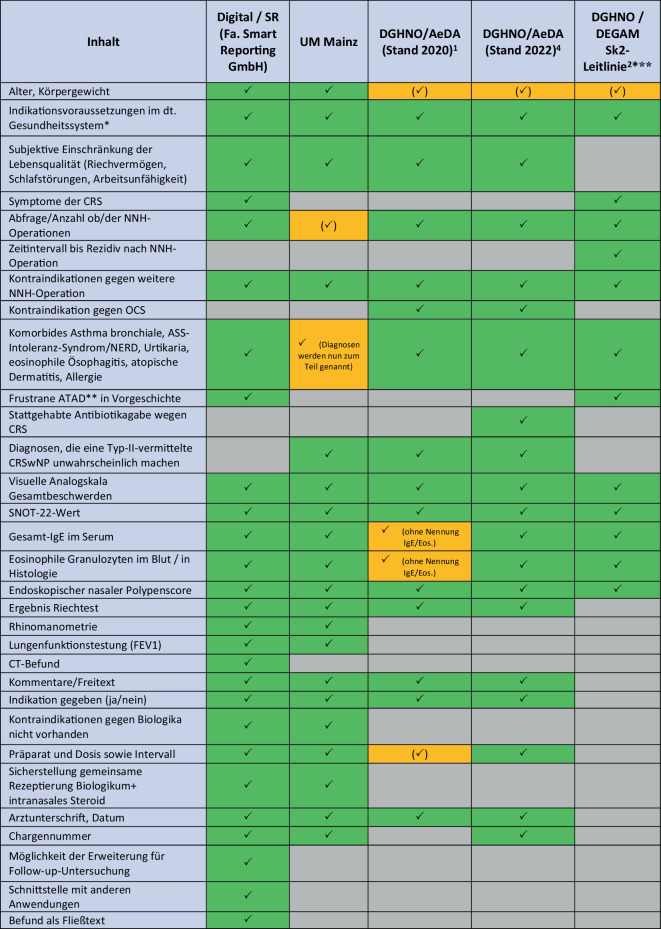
Unterschiede zwischen den Dokumentationsmöglichkeiten.  *Diagnose, schwere Erkrankung, Z. n. oralen Kortikosteroiden (*OCS*) und/oder Nasennebenhöhlen(*NNH*)-Operation, fehlende Krankheitskontrolle unter topischen Steroiden;  *ATAD*: adaptive Desaktivierung mit Acetylsalicylsäure (*ASS*); * zum Zeitpunkt der Studie noch nicht verfügbar. *Grün *vorhanden. *Orange und Häkchen in Klammern *mit Einschränkung vorhanden. *Grau* nicht vorhanden. *AeDA *Ärzteverband Deutscher Allergologen e. V.*; CRS *chronische Rhinosinusitis*; CRSwNP *chronische Rhinosinusitis mit Polyposis nasi; *CT* Computertomographie; *DEGAM *Deutsche Gesellschaft für Allgemeinmedizin und Familienmedizin*;*
